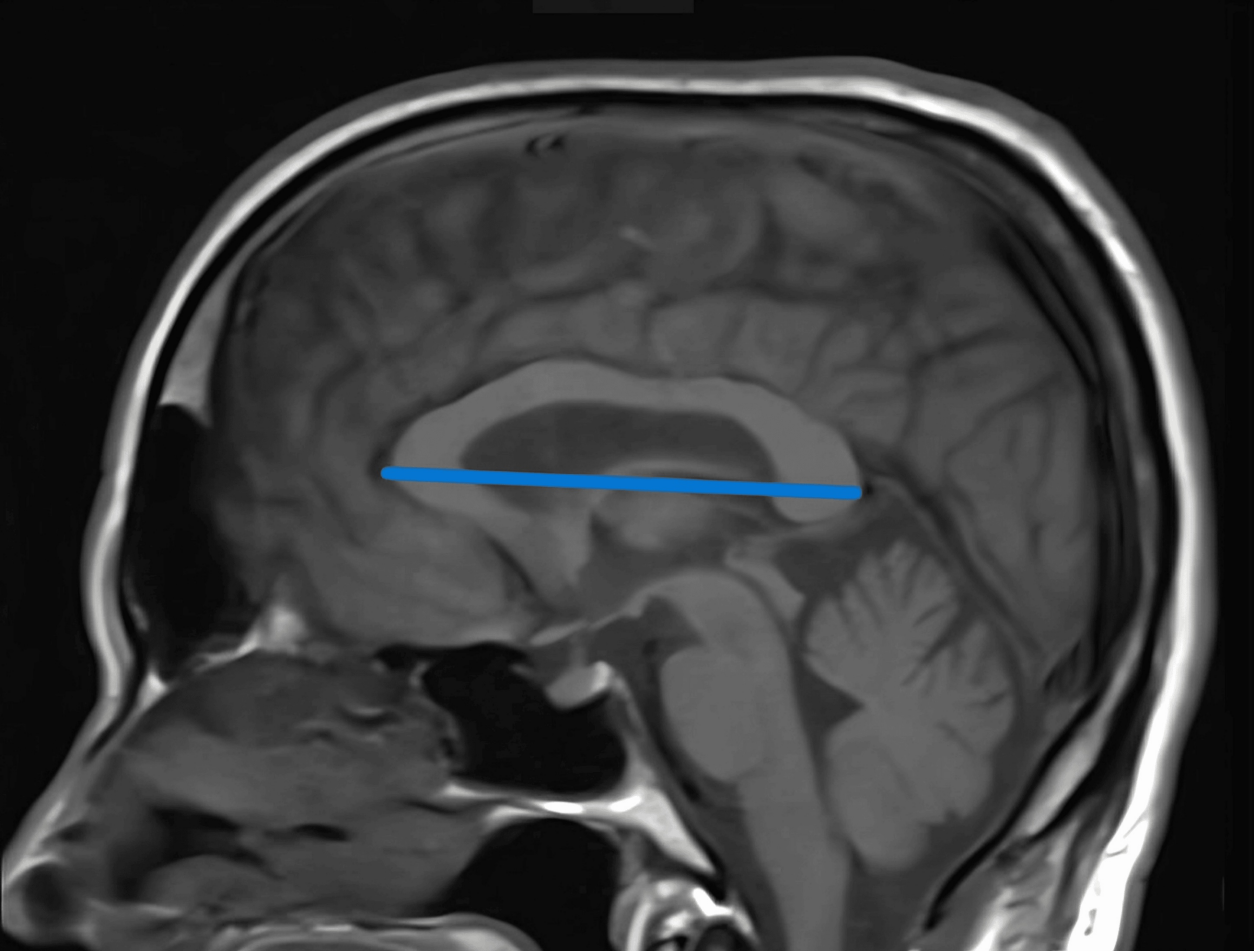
Mid-sagittal T1-weighted MR image showing the measurement of the anteroposterior diameter of the corpus callosum: distance between the anterior aspect of the genu and the posterior aspect of the splenium
